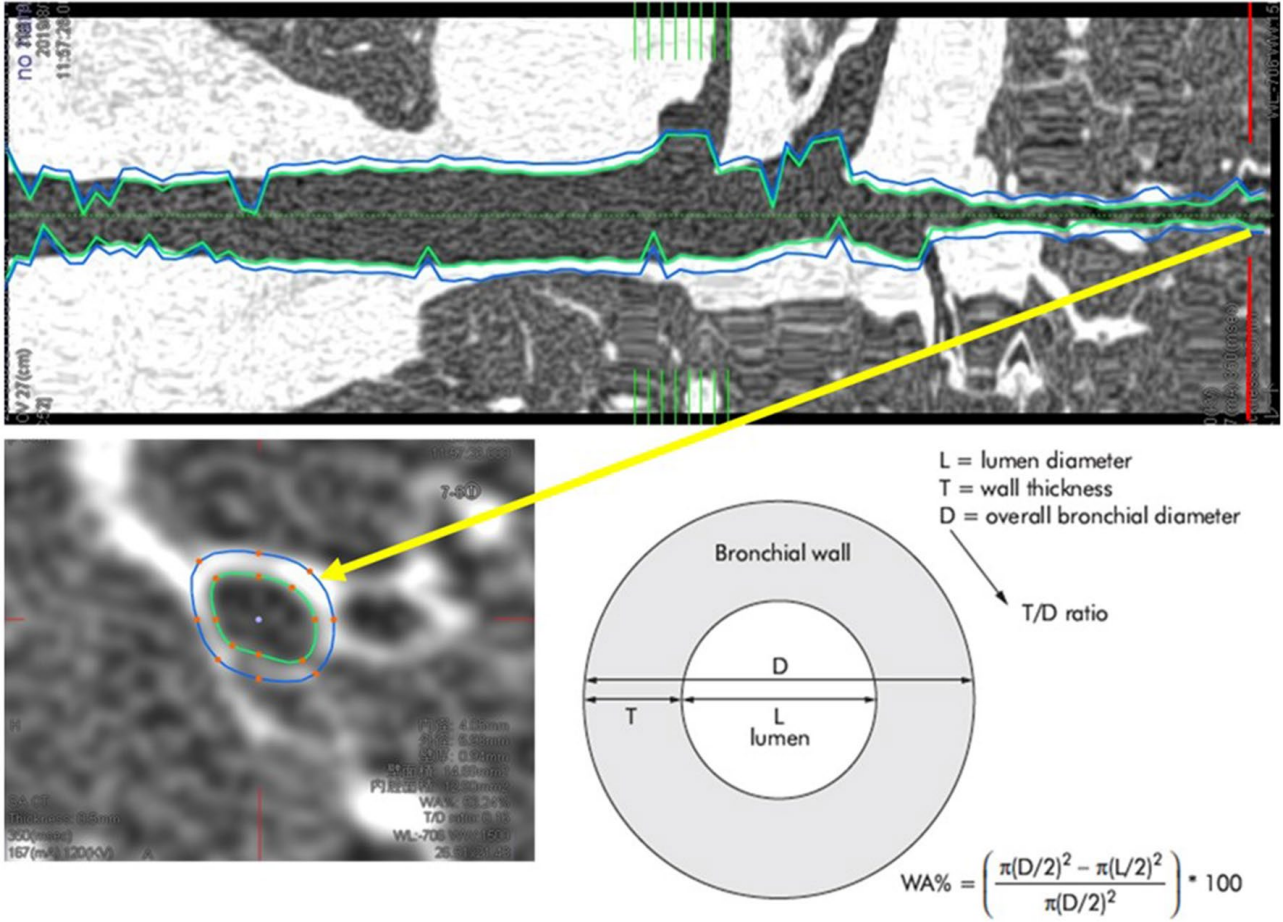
Measurement procedure using a CT-3D imaging workstation. The bronchus to be measured was selected on the left CT image, and a bronchial pathway was created by automatic extraction (*top*). The bronchial cross section at the specified position is displayed and the inner and outer diameters are marked (*bottom left*). The inner and outer diameters, WA%, and T/D ratio were automatically calculated for the 4th-8th generations of bronchi (*bottom right*). L: lumen diameter, T: wall thickness, D: overall bronchial diameter. In the measurement screen, multiple measurements of T, D, and L are made, and their average are calculated
